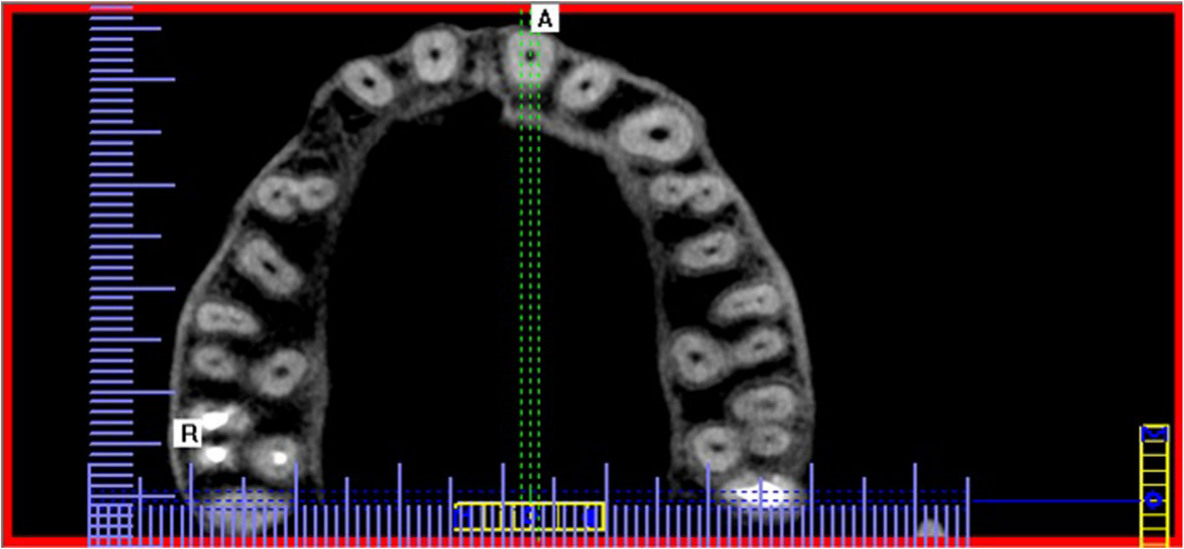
Cross-sectional CBCT image of maxillary first and second molars showing four root canals and three roots
